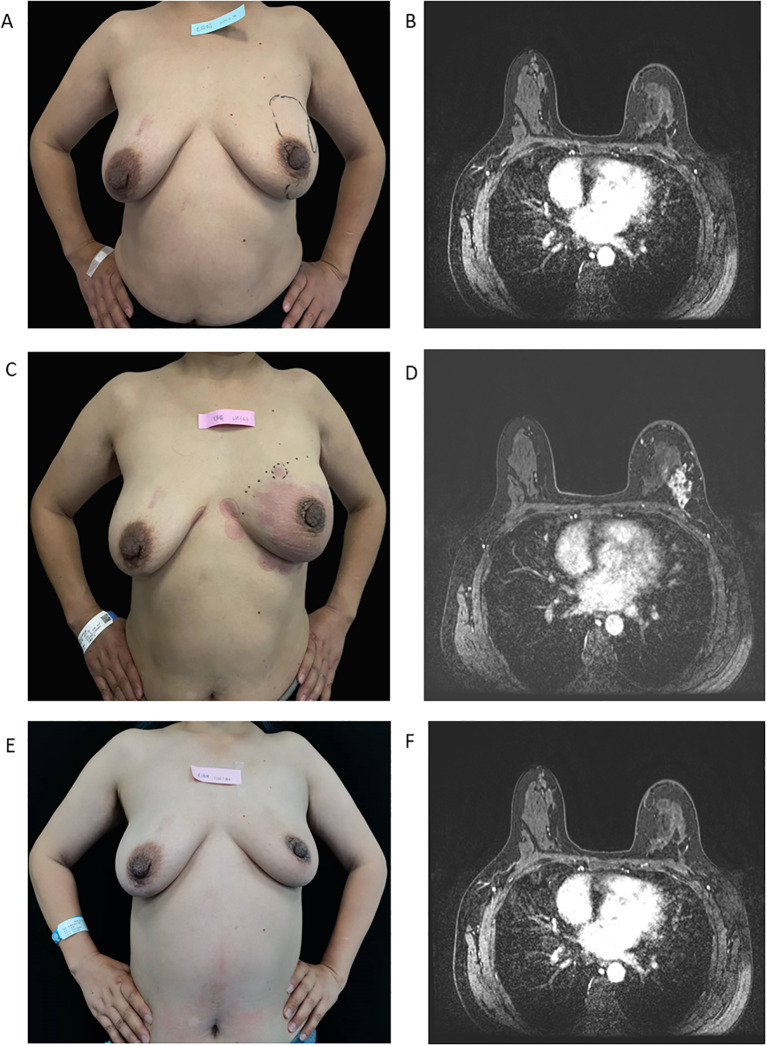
Treatment response assessment by serial breast photographs and MRI. (A, B) Breast photographs and MRI after four cycles of docetaxel plus trastuzumab plus pyrotinib, showing resolution of left breast erythema, improvement in edema, and reduction in the extent of the lesions. (C, D) Breast photographs and MRI after ten cycles of docetaxel plus trastuzumab plus pyrotinib, showing recurrence of erythema and increased lesion extent compared with the prior assessment, consistent with disease progression. (E, F) Breast photographs and MRI after four cycles of T-DXd, showing improvement of erythema and edema with a reduced extent of the lesions.
